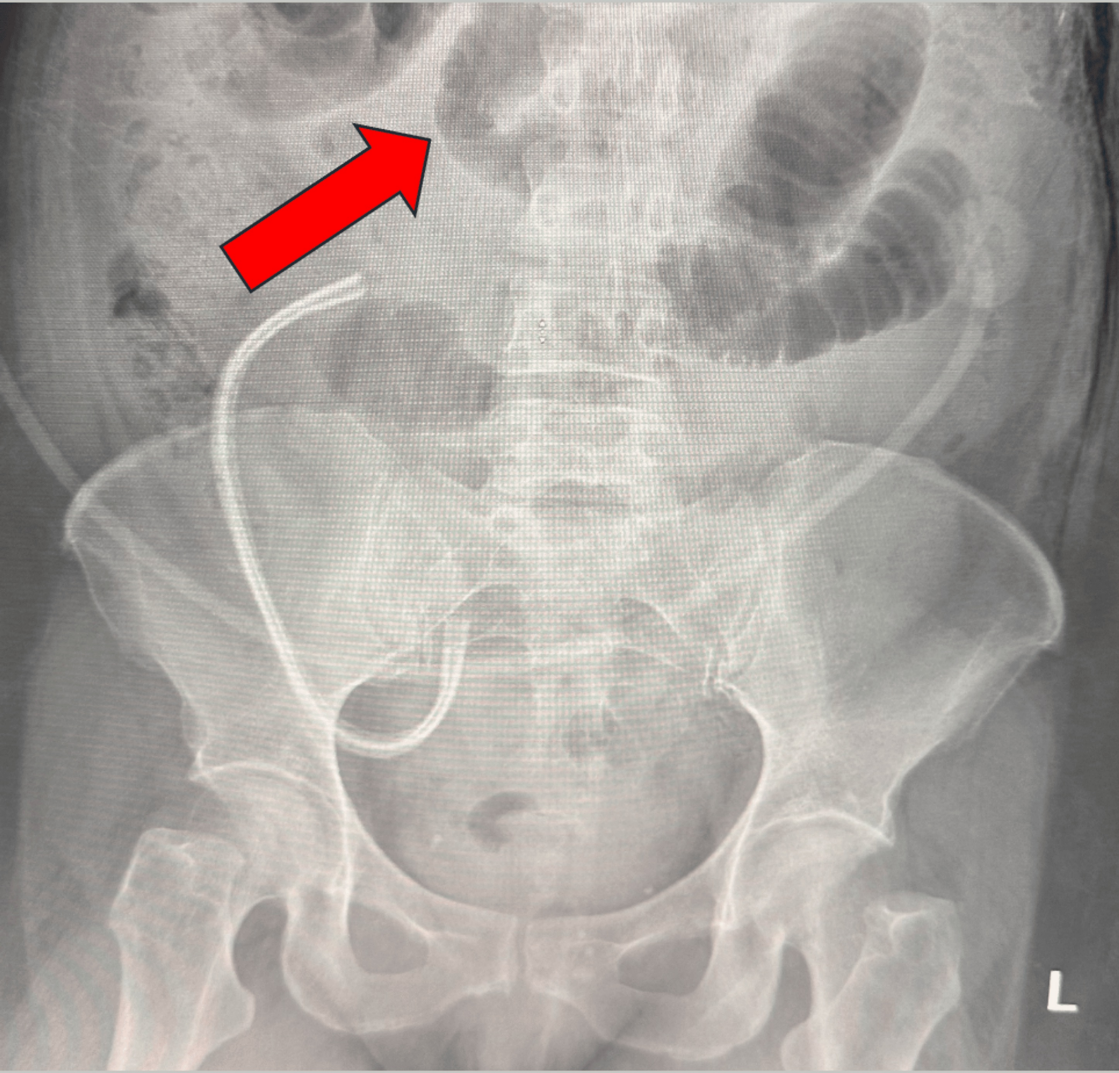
KUB radiograph showing diffuse small bowel dilation consistent with a postoperative ileus. Arrow size: 3.2 cm x 0.8 cm KUB: Kidney, urinary, bladder
